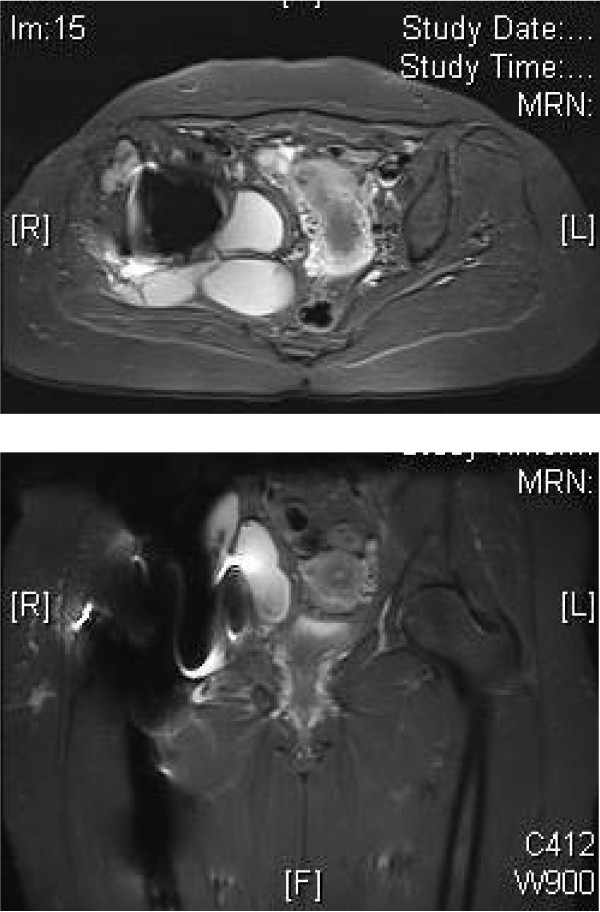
MRI scans showing recurrent Hydatid cysts.
